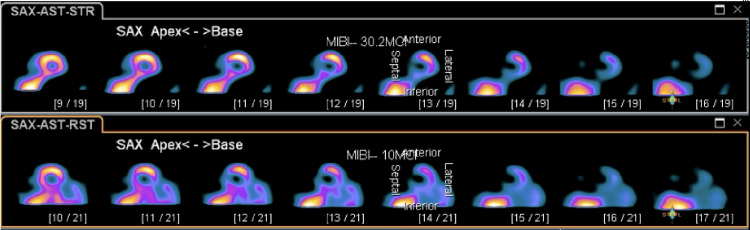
SPECT image 1 showing a small to moderate reversible perfusion defect present in the mid and basal aspect of the inferoseptal wall of the left ventricle. SPECT: single photon emission computed tomography.
